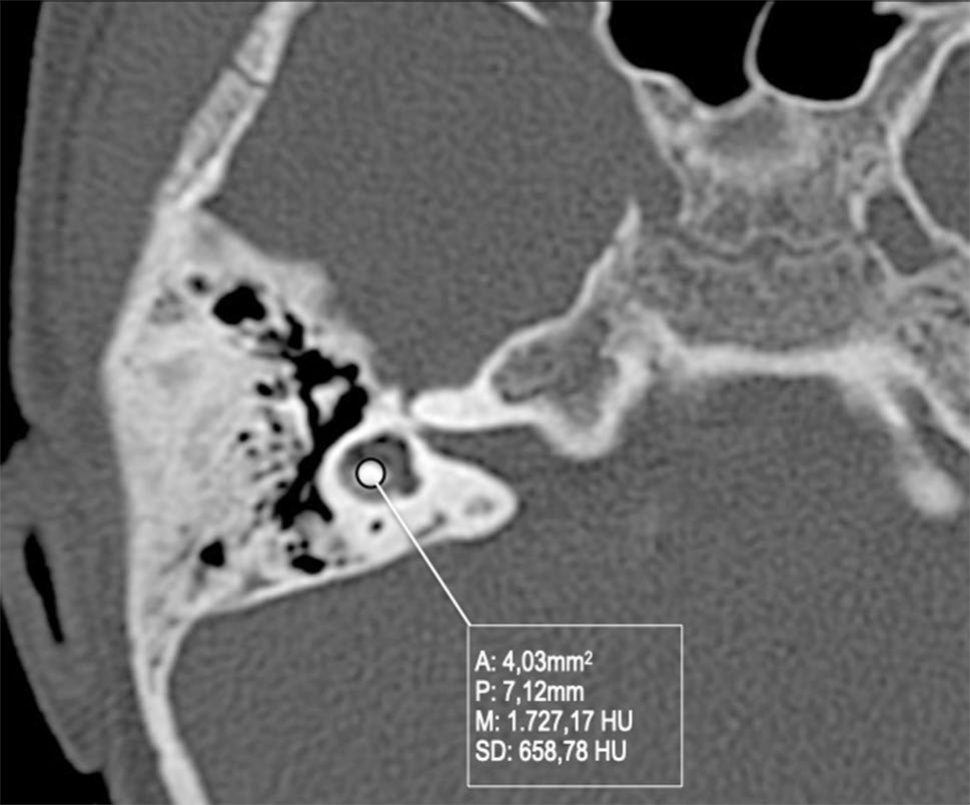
A 3-year-old female with right progressive SNHL. Axial CT obtained at IAC level shows an enlarged vestibule. Note that the bone island of the lateral SCC is below 6mm^2^ (measured 4.03 mm^2^)
